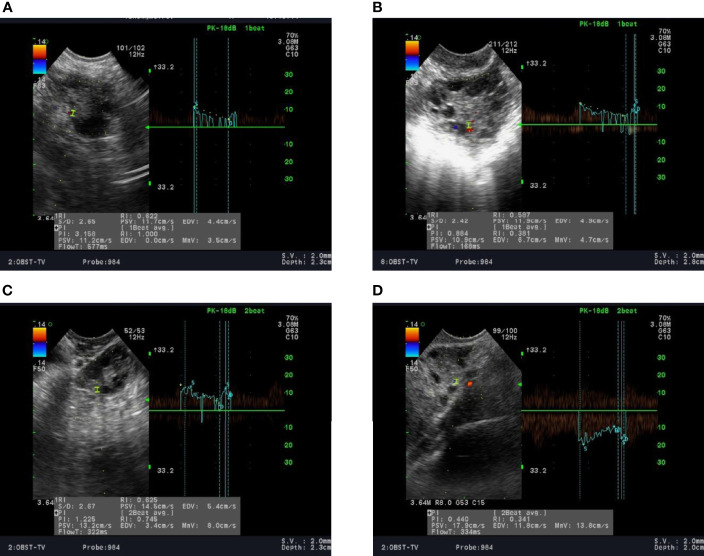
Ultrasound recording of the ovarian blood flow in: (A) a normal female; (B–D) in patients with PCOS stratified into phenotypes A, B, and D, respectively, as described in the methods section.
